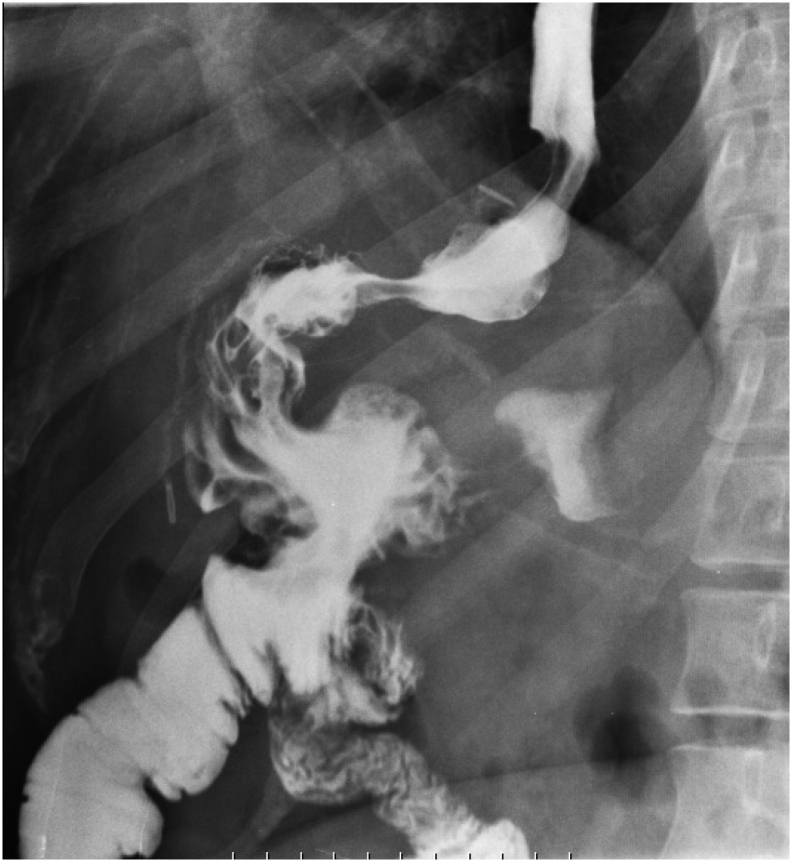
Barium swallow and meal radiography revealed normal gastroesophageal tract.
